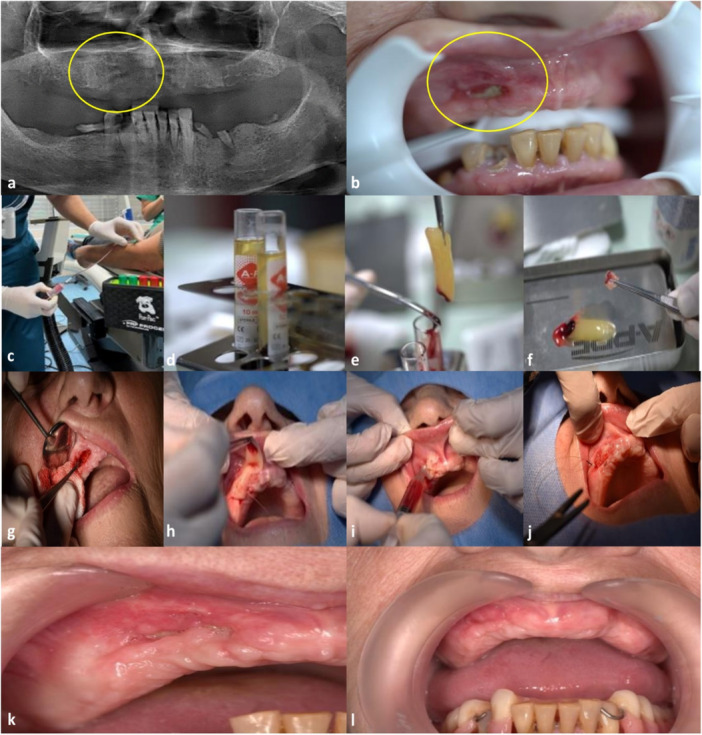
Case 4. A 69‐year‐old woman. (a) Orthopantomogram (OPG) obtained prior to A‐PRF application. (b) Nonhealing osteonecrotic lesion located in the right anterior maxillary region. (c–f) Preparation of A‐PRF: venous blood collection, A‐PRF tube after centrifugation, fibrin clot formation, and shaping of the A‐PRF clot. (g) Surgical resection of infected and necrotic tissues. (h) Placement of the shaped A‐PRF clot and coverage with a PRF membrane. (i) Initial application of liquid PRF. (j) Wound closure with sutures. (k) Healed surgical site 1 month after the first A‐PRF application. (l) Stable healing observed 1 year after the first A‐PRF application.
